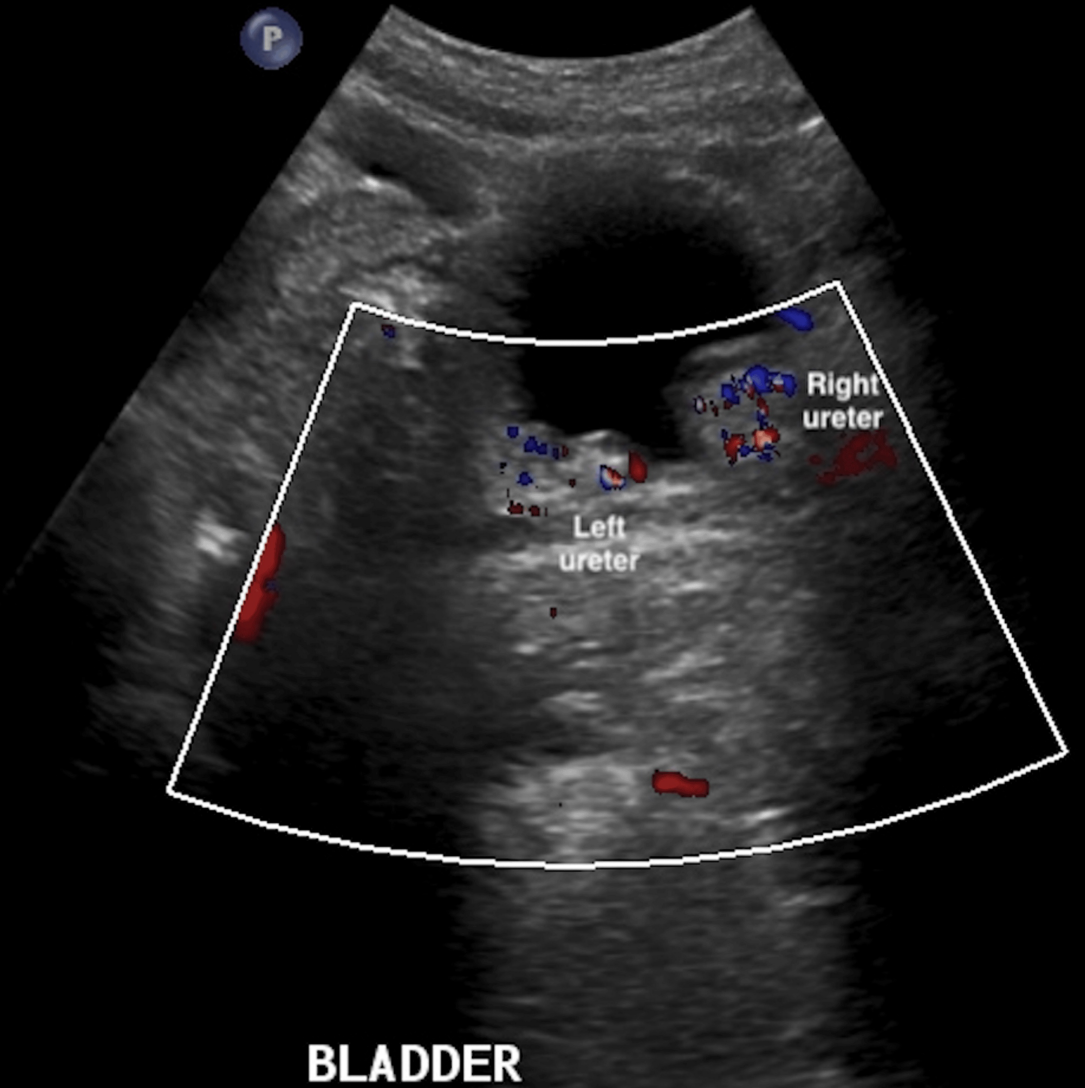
The ultrasound revealed bilateral ureterovesical junction obstruction, with both ureters contrasting at the lower level when applying the Doppler tool.
